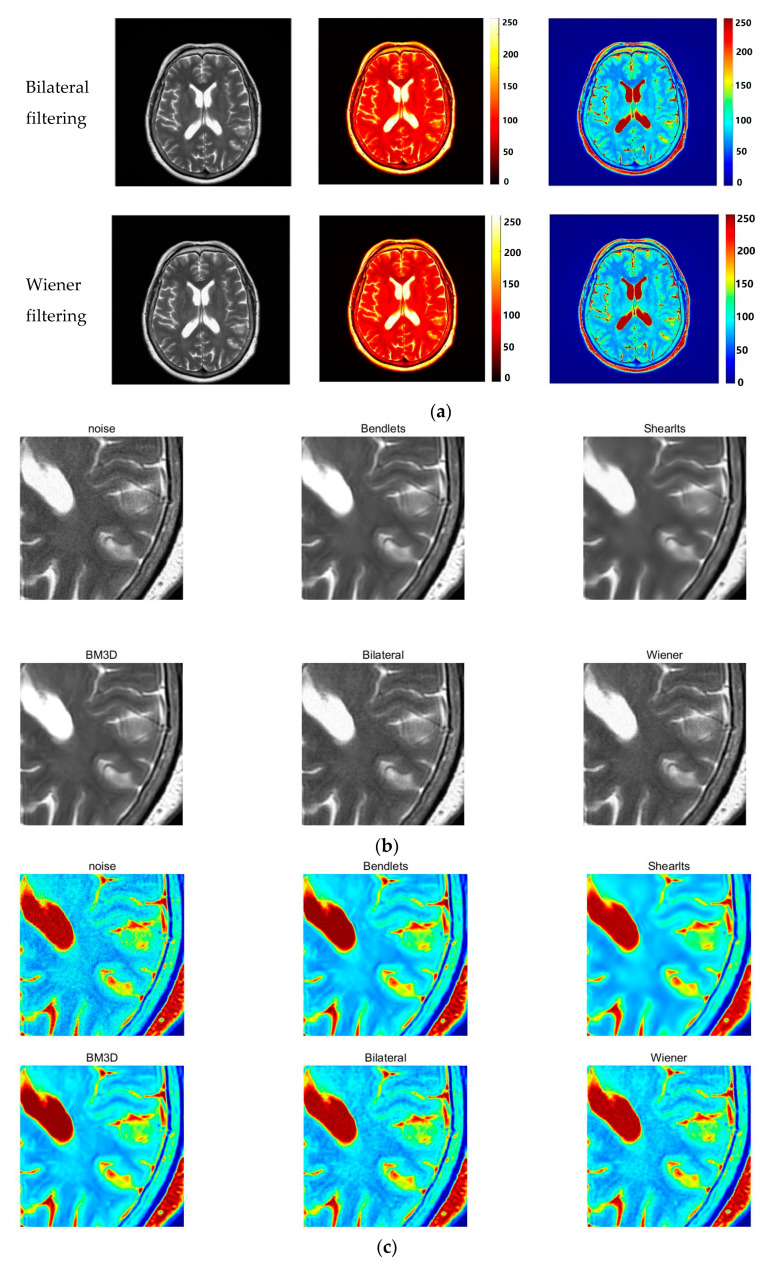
Comparison of denoising methods for MRI with noise. (a) Comparison of MRI noise reduction images with different methods. (b) Gray scale image of partially amplified MRI denoising by different algorithms. (c) Jet image denoising of partially amplified MRI by different algorithms.
